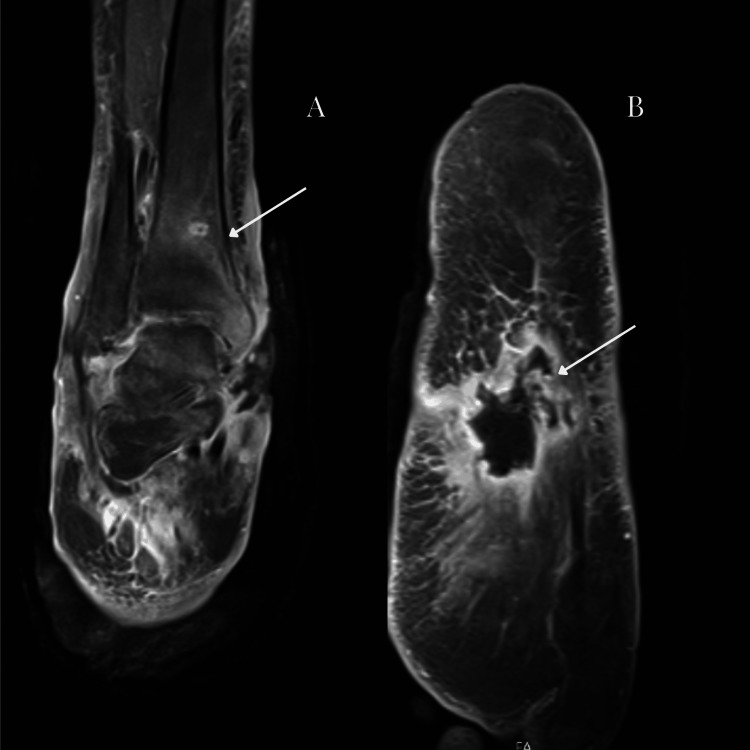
Right foot magnetic resonance imaging (osteomyelitic lesions) A) Area of cutaneous ulceration with a fistulous tract, associated with a pronounced adjacent inflammatory process, accompanied by thickening of the posterior tibial tendon and erosion at the medial region level, B) Dysmorphia with ankylosis and erosions of the bony elements of the midfoot, in relation to changes in the context of chronic osteomyelitis
